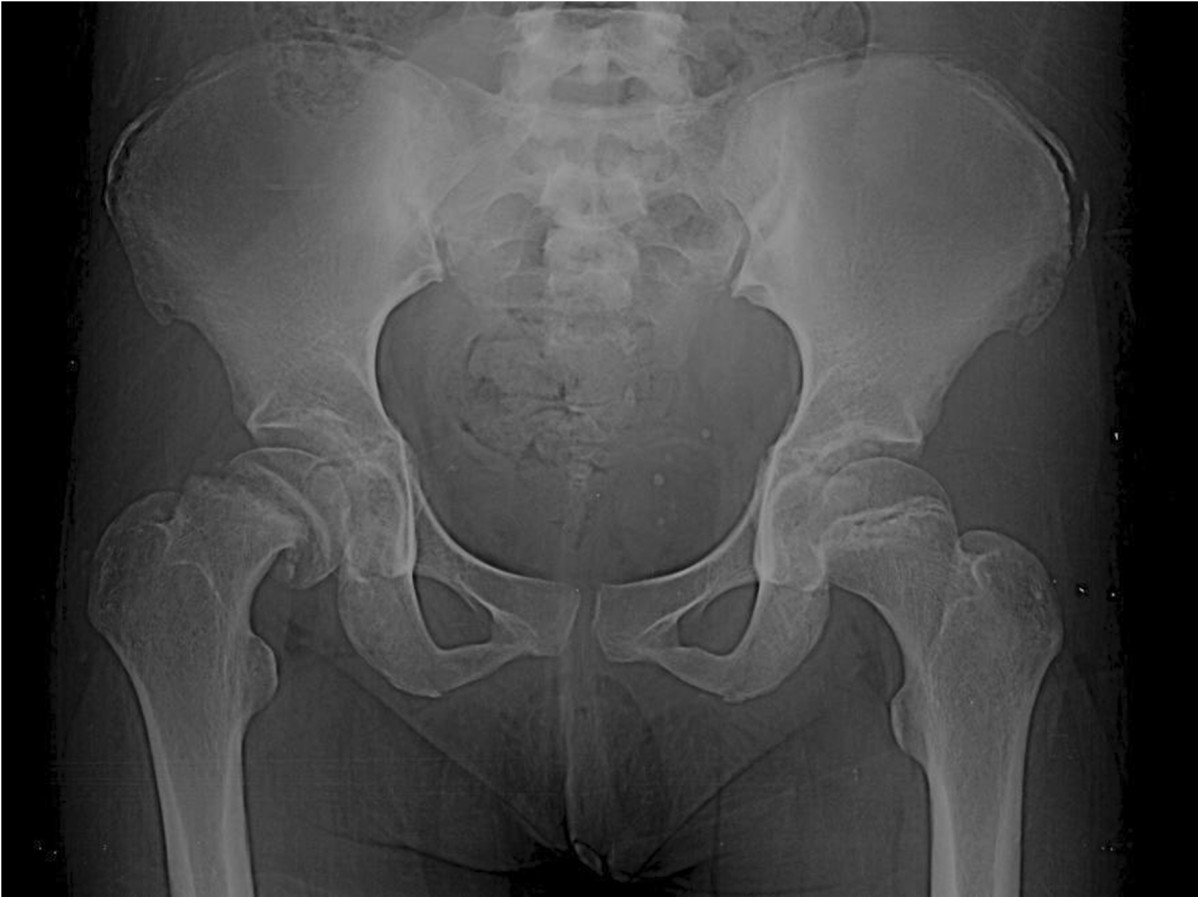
Pre-operative radiograph showing moderate-grade slipped capital femoral epiphysis and Risser grade IV calcification.
